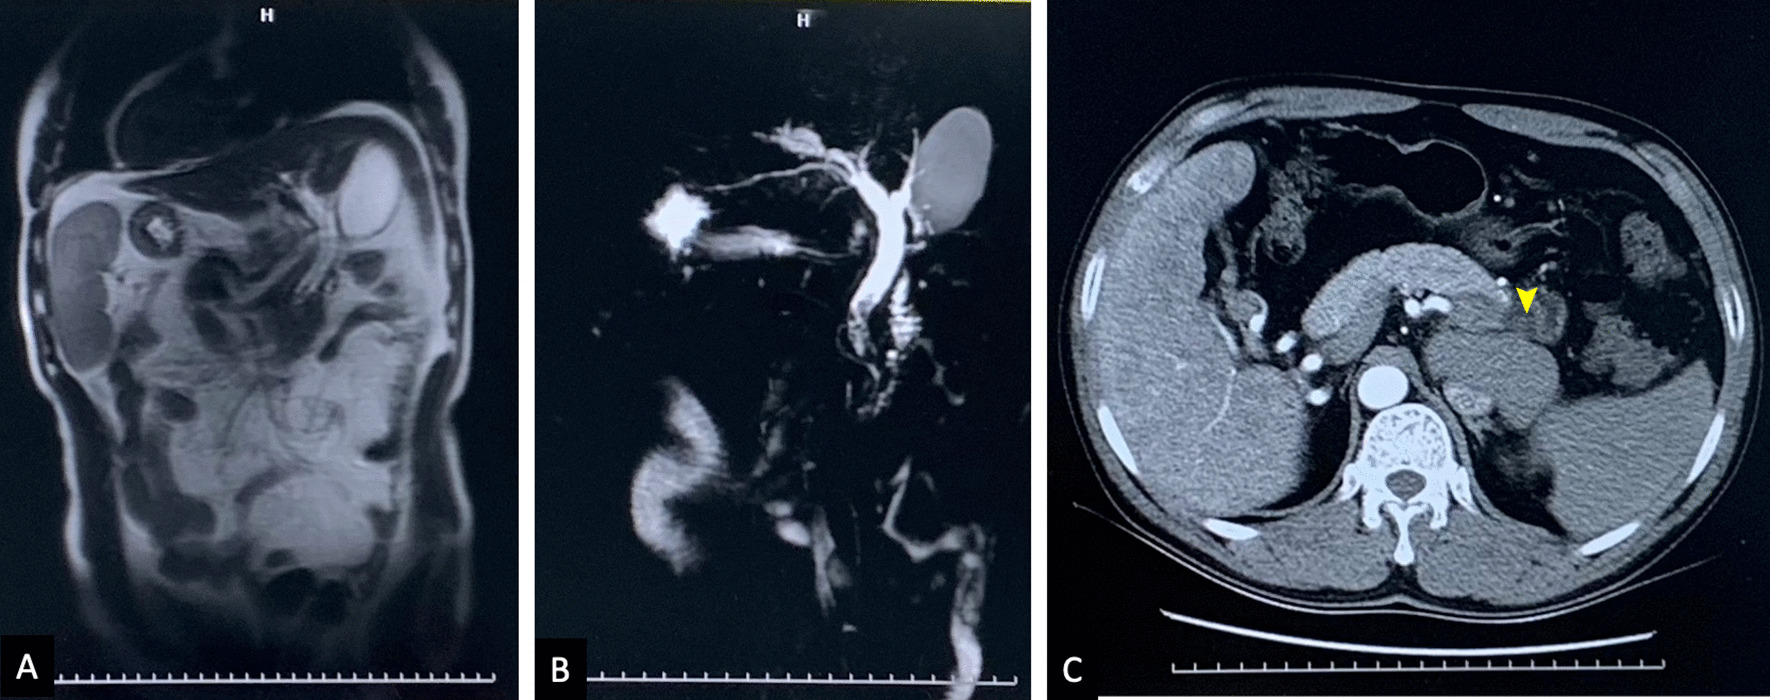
MRI/MRCP and CT images of a SIT patient with CBDS and decompensated cirrhosis. A. coronal MRI image shows the mirror-image transposition of abdominal and thorax viscera. B. reconstruction image indicates multiple small stones piled inside the middle-distal of CBD, the conference of bile duct and pancreatic duct seems located in the lower corner or the horizontal part of duodenum. C. CT image of the SIT patients, with yellow arrowhead points to the hypodense or isodense biliary stones
